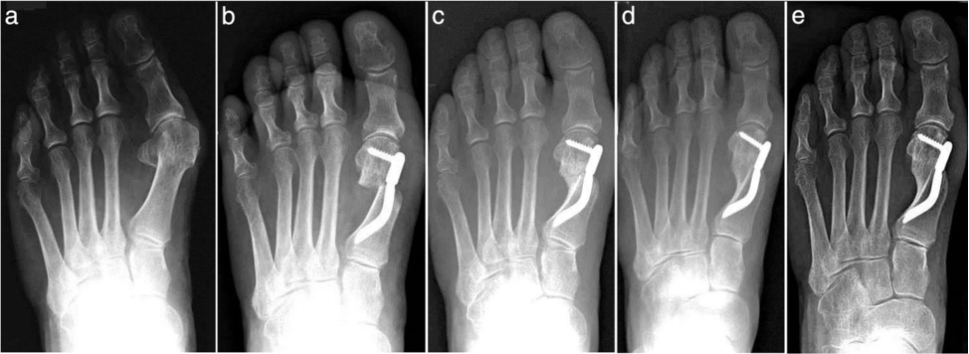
Case 2. A 64-year-old woman with moderate HV; antero-posterior radiographic images at: a Preoperative period. b 1-month follow-up. c 3-month follow-up. d 6-month follow-up. e 48-month follow-up showing the maintained correction of the deformity
